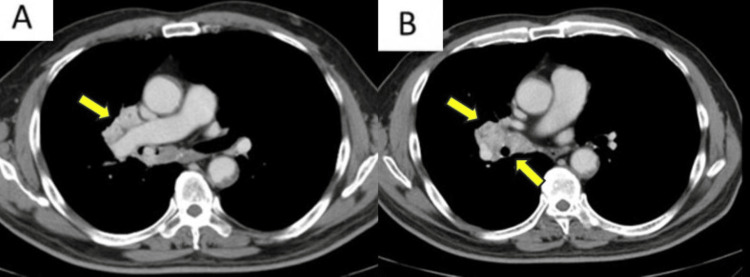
(Panel A) contrast-enhanced chest computed tomography revealing a heterogeneous tumor (yellow arrows) extending from the tracheal bifurcation to the right hilum, and (Panel B) causing stenosis of the right main bronchus
